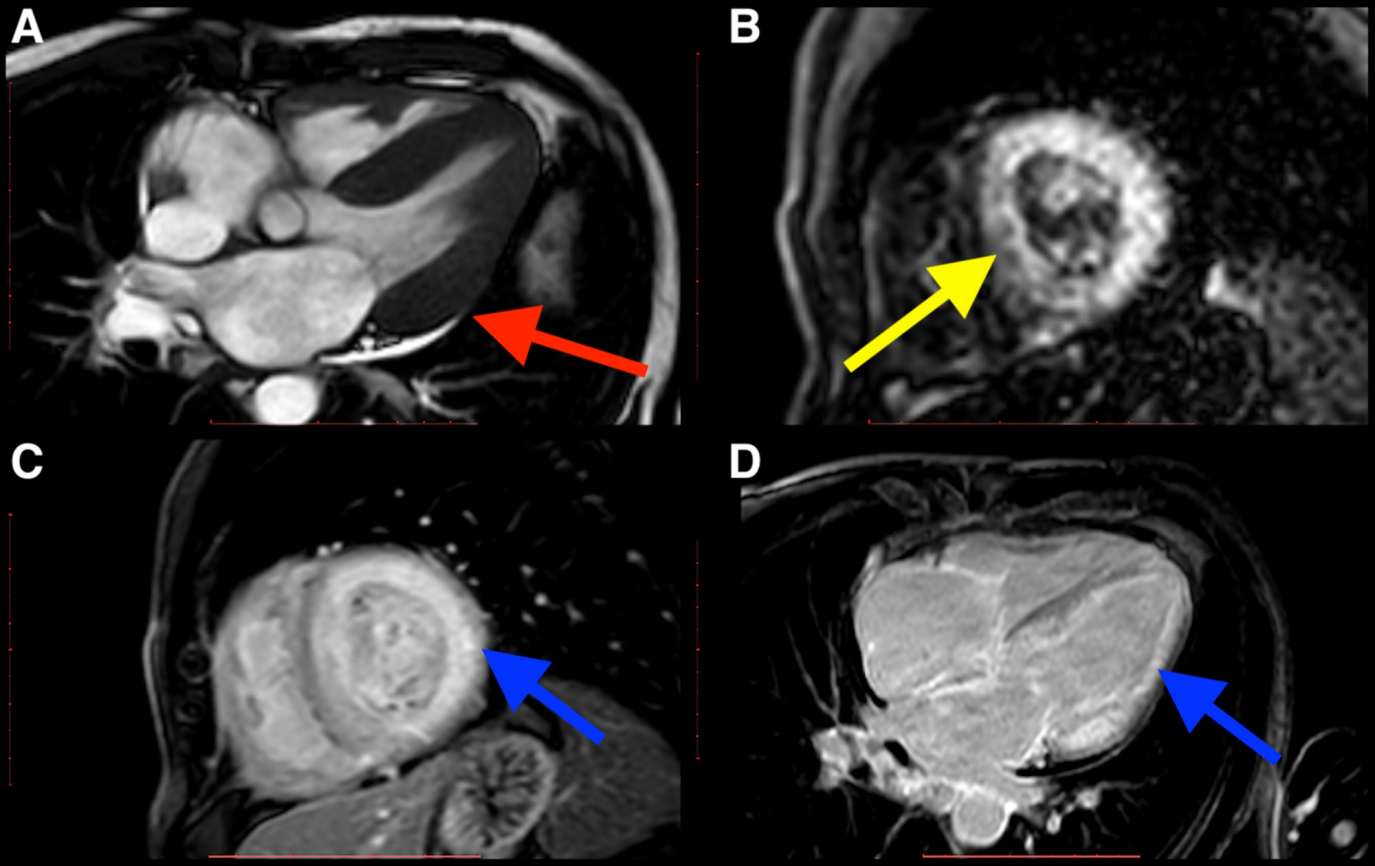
Cardiac magnetic resonance imaging of an amyloidosis patient. A four-chamber cine image depicts concentric moderate-to-severe left ventricular hypertrophy with small pericardial and bilateral pleural effusion (red arrow in A). On IR-TFE imaging, myocardial nulling could not be achieved (yellow arrow in B). In C and D, there is diffuse late gadolinium enhancement of the left ventricle, both atria and the right ventricle myocardium (blue arrows). IR-TFE images: inversion conversion-turbo field echo images.
